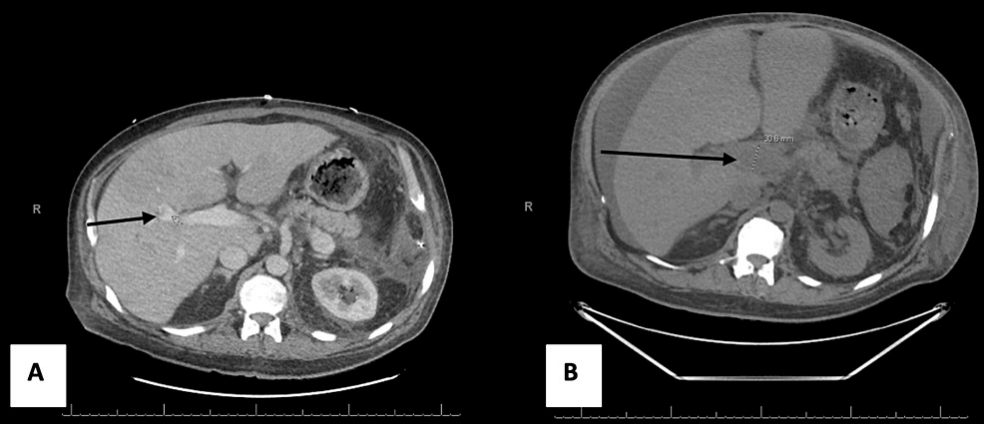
This figure compares two axial views of CT scans of the pulmonary abdomen pelvis taken in January 2023 (A) and April 2023 (B). In B, the thrombus within the branch of the right portal venous system clearly extends into the anterior segment. The progression of the portal vein thrombosis over the three-month period can be seen between A and B.
